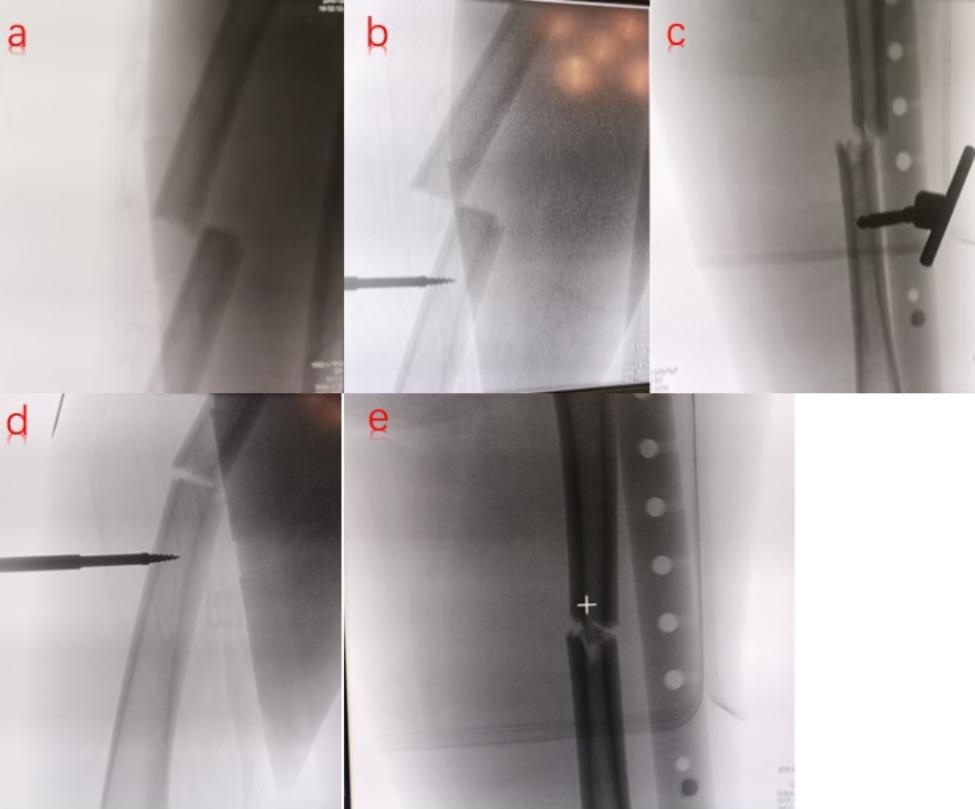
Reduction of fractures in the middle and lower femoral shaft by DRTR: (a) Lateral radiographs of the fracture before reduction; (b) Lateral radiographs of the distal femoral fracture inserted with a Kirschner steel needle; (c) Anterior radiographs of the distal femur fracture inserted with a Kirschner steel needle; (d) Lateral radiographs of the femur after reduction; (e) Anteroposterior radiograph after reduction
